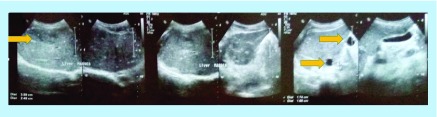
Ultrasonography showing multiple hepatic lesions after two cycles of doxorubicin monotherapy.
